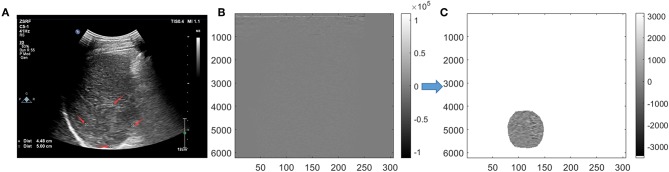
Tumor segmentation. On grayscale ultrasound image, the region of interest (ROI) was manually marked by a doctor with four white forks points (A). Image were segmented in scan-line imaging way for original radio frequency (ORF) data (B). After Hilbert transform and logarithmic compression of ORF signals, the grayscale ultrasound images under the scan-line images could be obtained (C).
